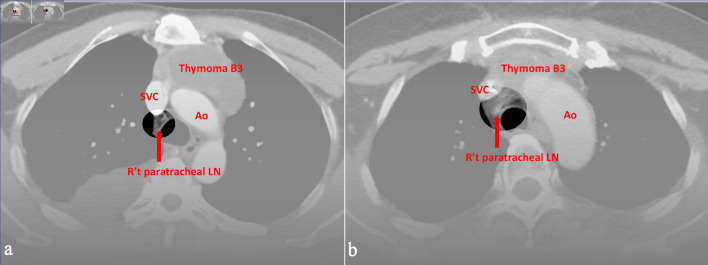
Preoperative CT images of (a) a 52-year-old male and (b) a 49-year-old female with World Health Organization (WHO) classification B3. All three radiologists evaluated right upper paratracheal LN metastasis, and the pathological report proved the positive findings. Ao: aorta; SVC: superior vena cava; CT: computed tomography; LN: lymph node.
